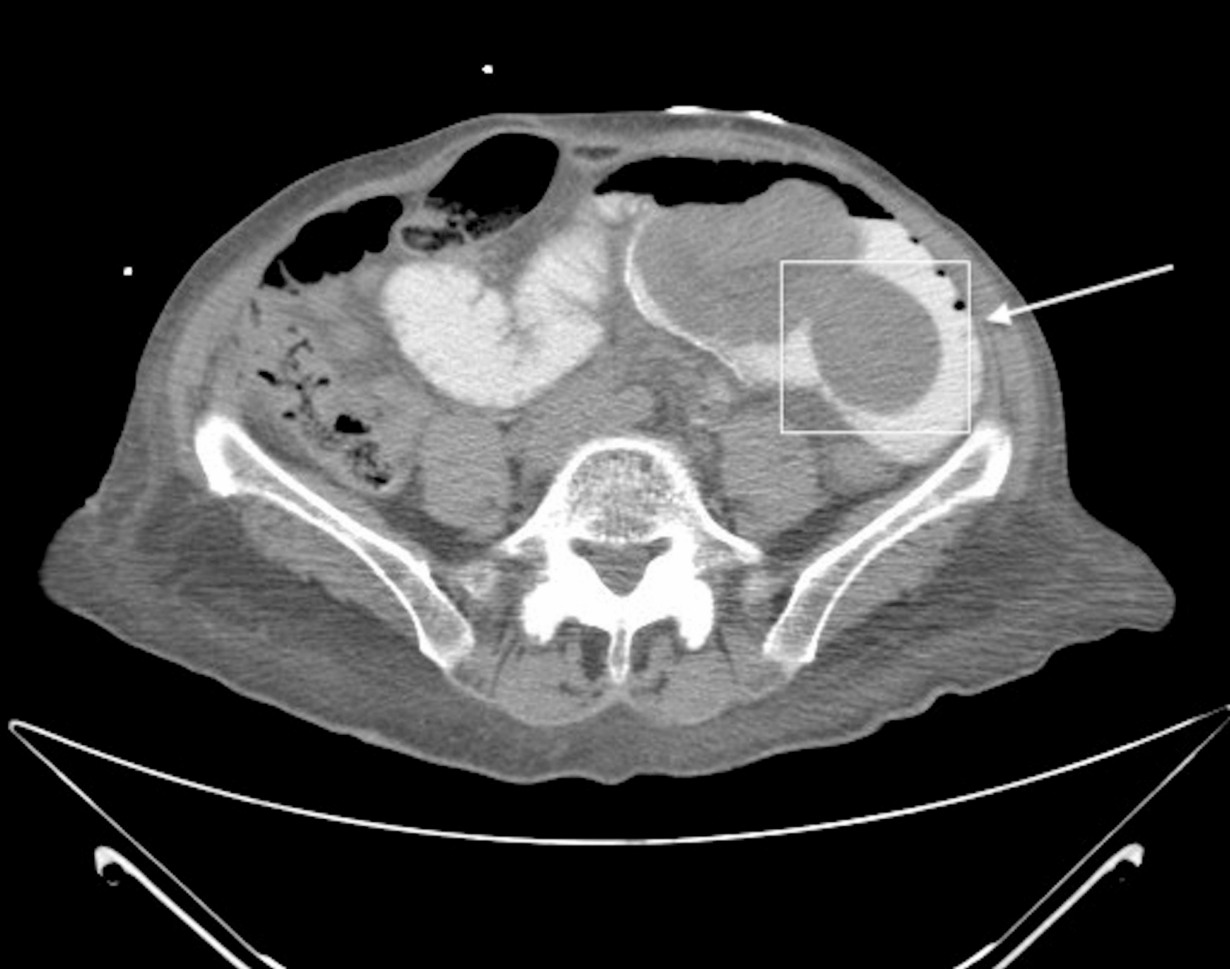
CT scan shows dilated small bowel loops involving left upper quadrant jejunal loops due to the intussusception CT: computed tomography
